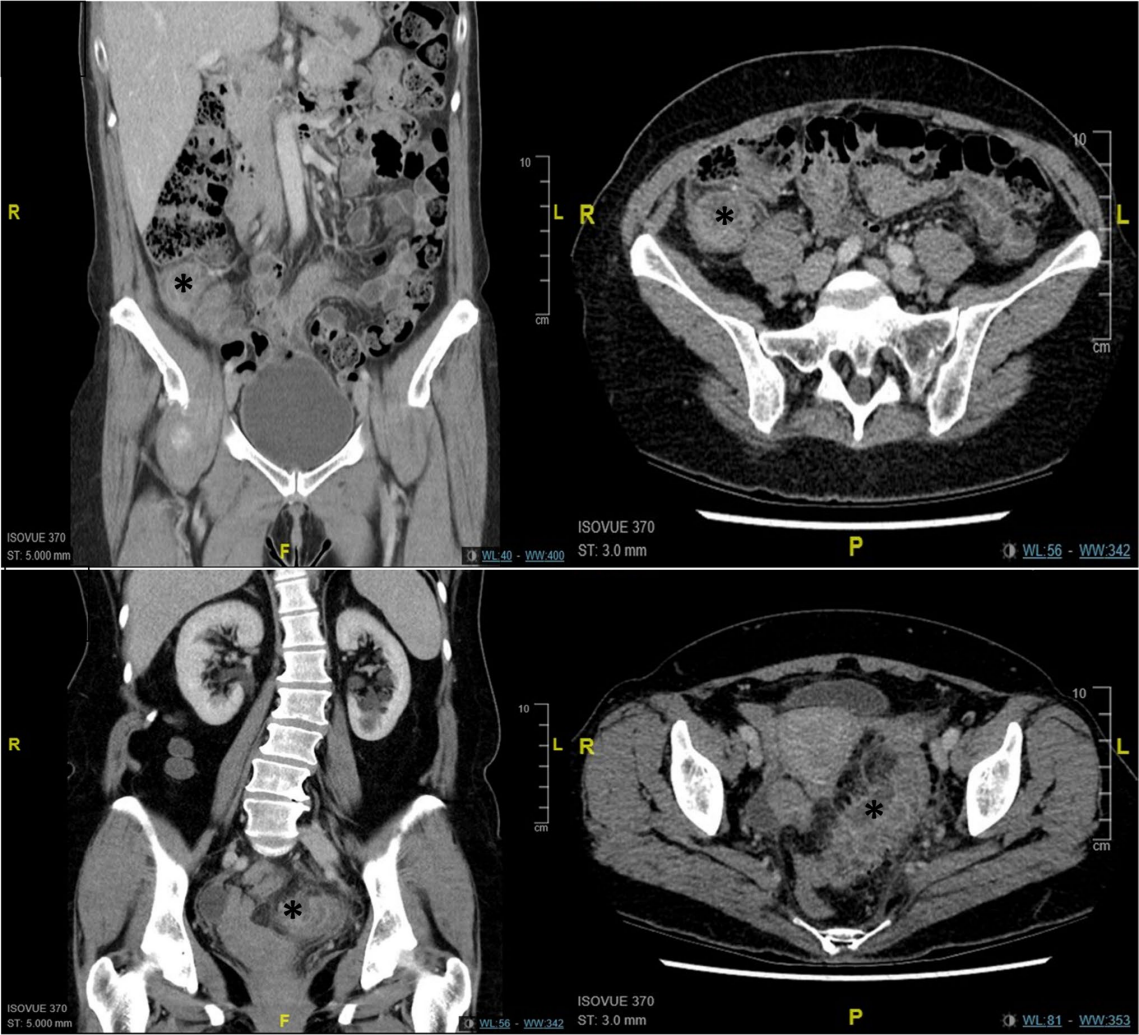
A Coronal and axial cut of a CT abdominal scan for patient with a complicated case of appendicitis with abscess demonstrated with an asterisk. B Coronal and axial cut of a CT abdominal scan for patient with a complicated diverticulitis with abscess demonstrated with an asterisk
